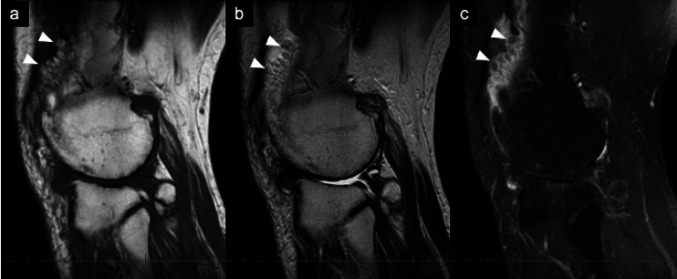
Lipoma arborescens. Sagittal T1-weighted (a) and T2-weighted (b) MR images show a frond-like mass within a suprapatellar bursa that has a signal intensity comparable to that of fat (arrows). Degenerative change of the knee joint is also seen. Sagittal fat-suppressed postcontrast T1-weighted image (c) shows marked gadolinium enhancement of the synovium excluding the fat mass (arrowheads)
